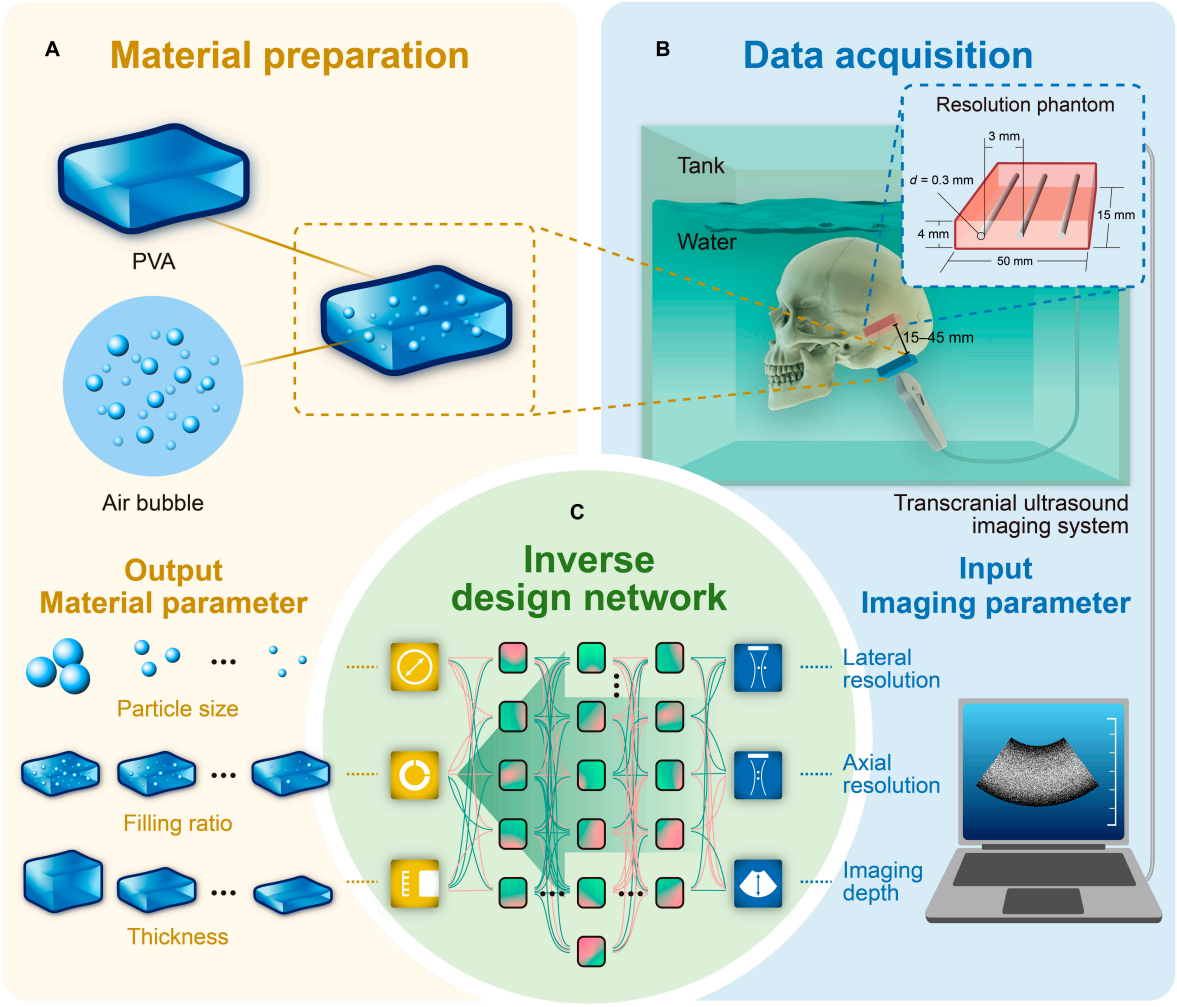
Neural-network-decision-based parameter prediction method for transcranial AMM. The inverse model inputs imaging parameters and predicts the corresponding material parameters. (A) The transcranial AMM preparation and the main parameter of the transcranial AMM. (B) The imaging parameter data acquisition from the transcranial ultrasound imaging system. (C) The reverse design approaches map the required transcranial ultrasound imaging system performance onto AMM parameters.
